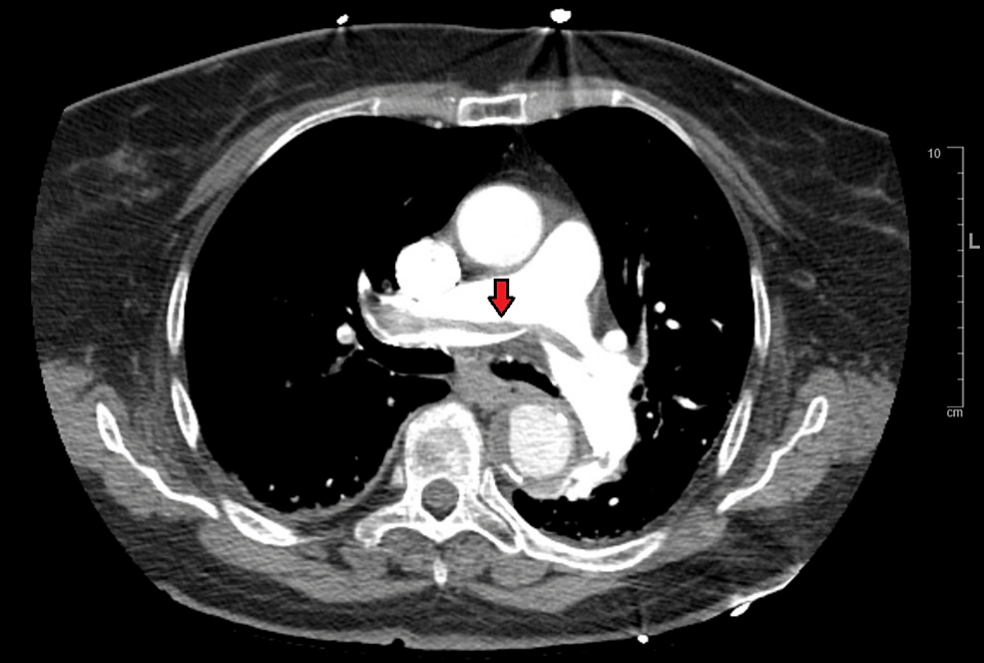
CT chest showing saddle pulmonary embolism (red arrow).
